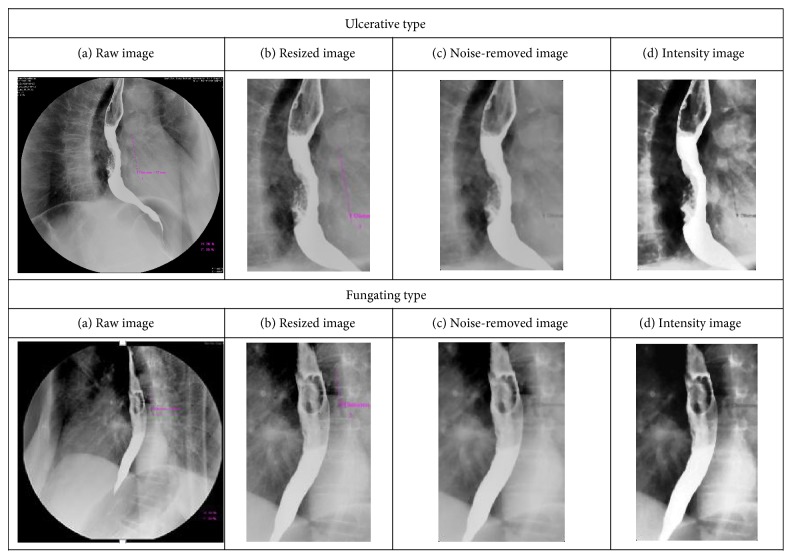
Preprocessing results of the abnormal esophageal X-ray images.
